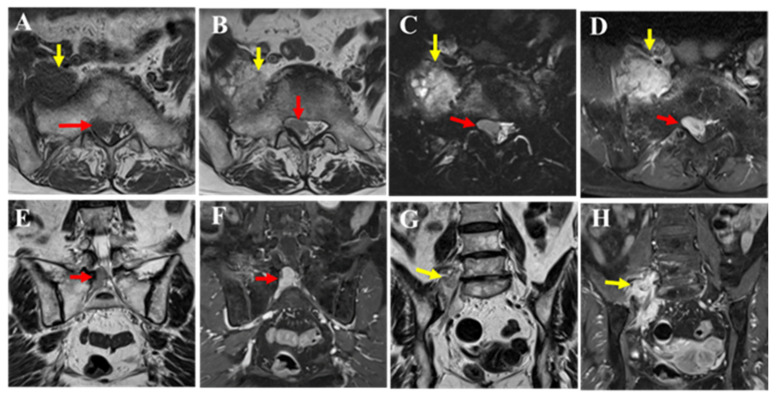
MRI revealed a mass extending from within the spinal canal at the L5/S1 level to the right intervertebral foramen (red arrow) and beyond the foramen (yellow arrow). The dural sac was displaced to the left of the spinal canal, indicating that the lesion was primarily extradural in nature. Compared to the muscle, the mass showed isointensity on T1-weighted images (A), mildly high signal intensity on T2-weighted images (B,E,G), and high signal intensity on T2 fat-suppressed images (C). Contrast enhancement was also observed (D,F,H). The portion of the mass outside the intervertebral foramen showed slightly heterogeneous internal characteristics on T2-weighted images (B,G yellow arrow), and nerve fibers were visible within it (G). Pathological findings from the CT-guided biopsy performed at the previous hospital suggested a spindle cell tumor. Although intermediate-to-low-grade malignancy was suspected, a definitive diagnosis could not be made. Therefore, an open biopsy was recommended to the patient; however, she declined, and the patient was followed conservatively. After two years, the patient experienced worsening pain and muscle weakness, leading to emergency hospitalization.
